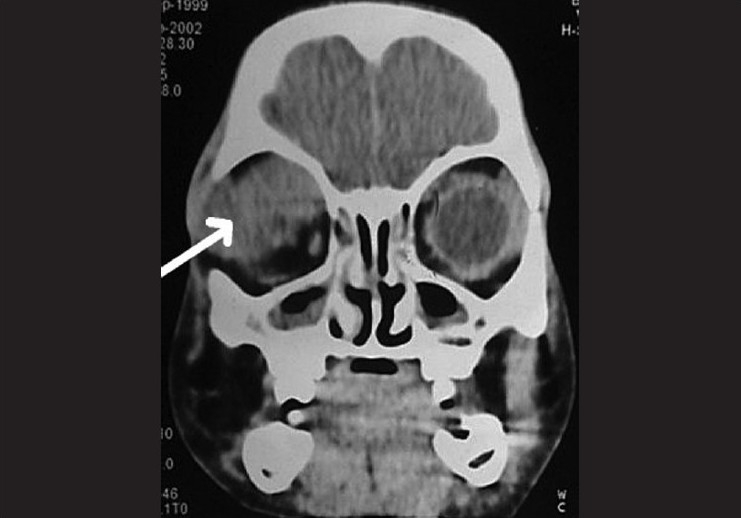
Coronal CT scan of the orbits showing a superior right orbital mass (arrow) displacing the globe inferiorly
